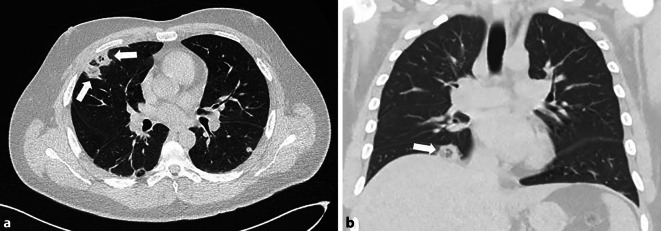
Native Computertomographie (CT) des Thorax im Lungenfenster mit axialen (a) und koronaren (b) Rekonstruktionen. Überwiegend peripher lokalisiert lassen sich im Mittellappen (a) und am Zwerchfell rechts im basalen Unterlappen (b) unscharfe Verdichtungen mit Lufteinschlüssen und umgebender Milchglastrübung abgrenzen (*Pfeile*)
